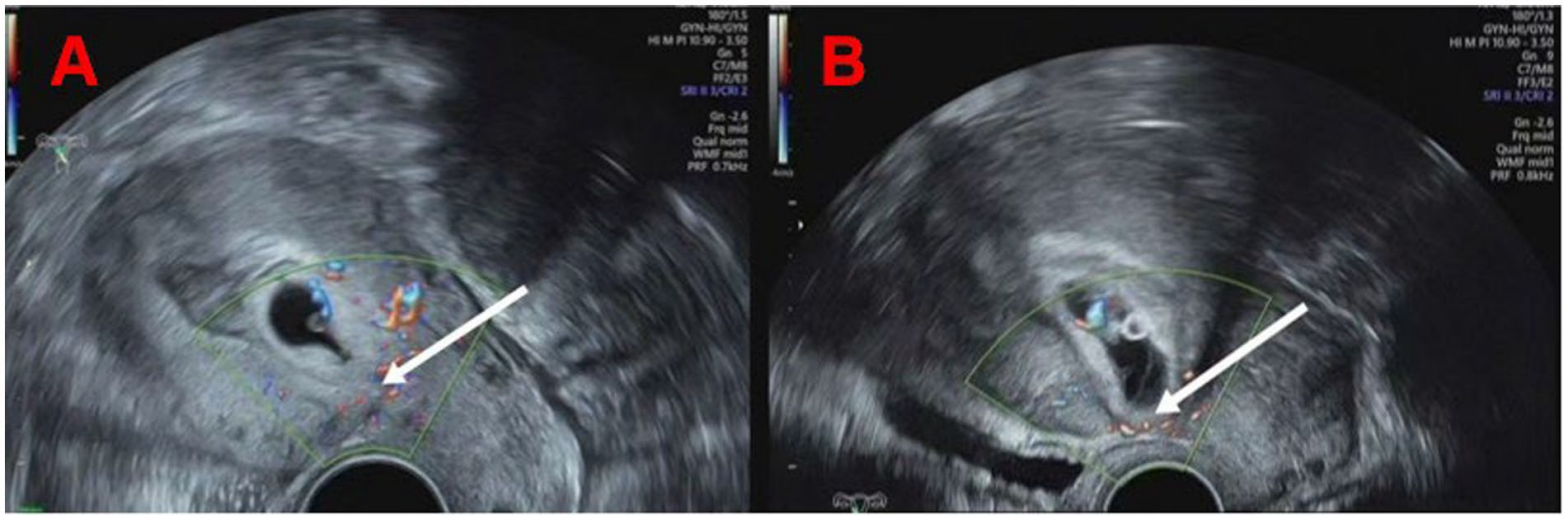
Typical color Doppler ultrasound images of type I and II CSP.
